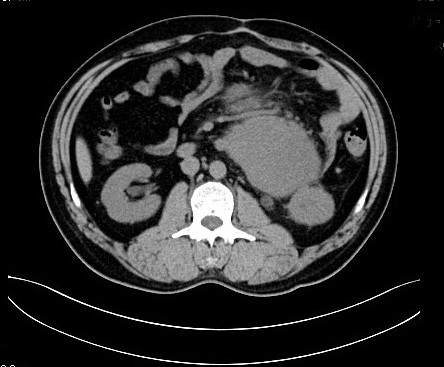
Preoperative CT scan images of the tumor. A contrast-enhanced CT image showing a homogeneous low-dense tumor with slight enhancement involving the proximal jejunum and descending colon.
